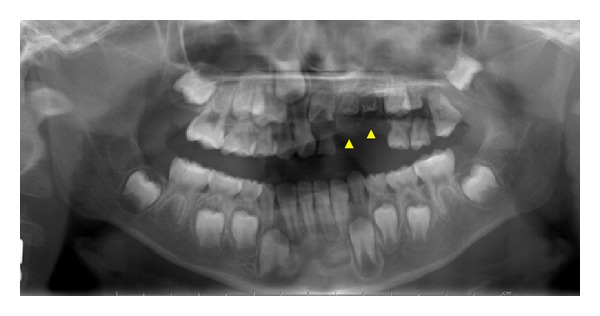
Panoramic radiograph at age 8 years and 4 months (case 1). Dental follicles of permanent affected teeth have irregularly expanded (arrowheads).
